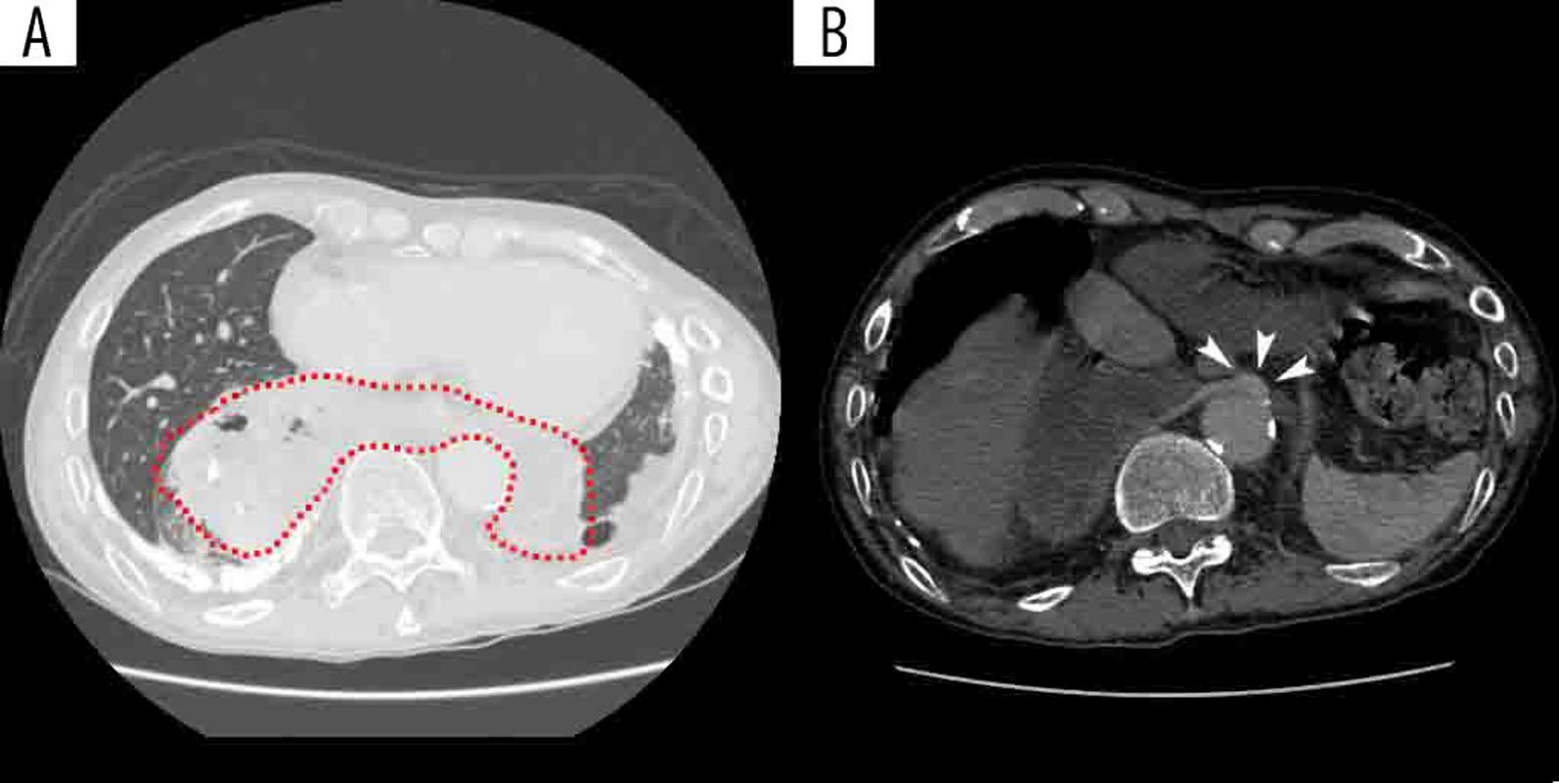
A Computed tomography showing the protruding lung from the right to the left side in front of the descending aorta and behind the esophagus (dotted line). B An abnormal blood vessel from the descending aorta to the lung is confirmed (arrow)
